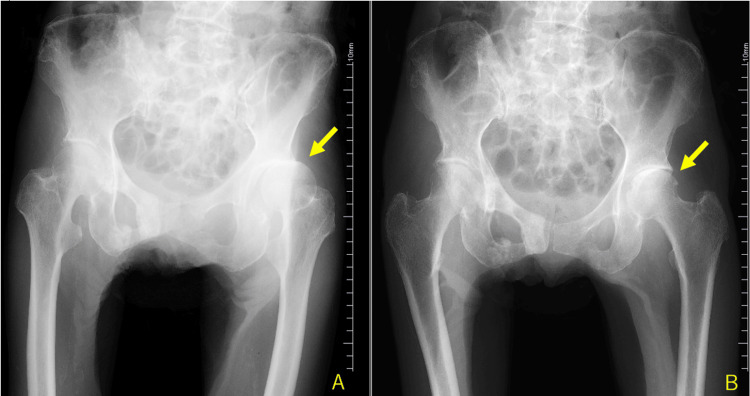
Plain radiographs of the hip joint. The dislocated left hip joint (A) was reduced very easily by internal rotation (B).
